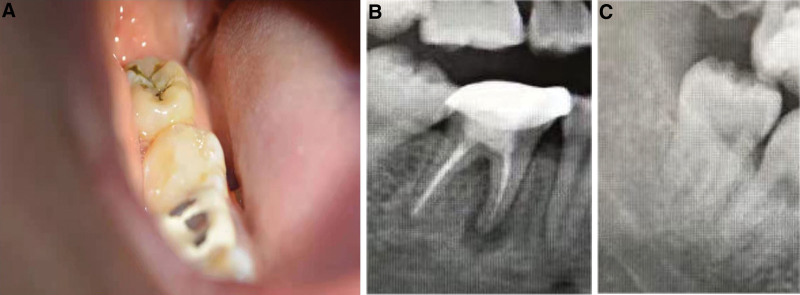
Imaging data before treatment: (A) clinical examination showed a poor PFM crown restoration on tooth 46; (B) preoperative panoramic radiographic indicating a severe peri-apical lesion invading the mesial root and the root furcation of tooth 46; (c) tooth 48 is intact and morphologically similar to tooth 46. PFM = porcelain fused to metal.
